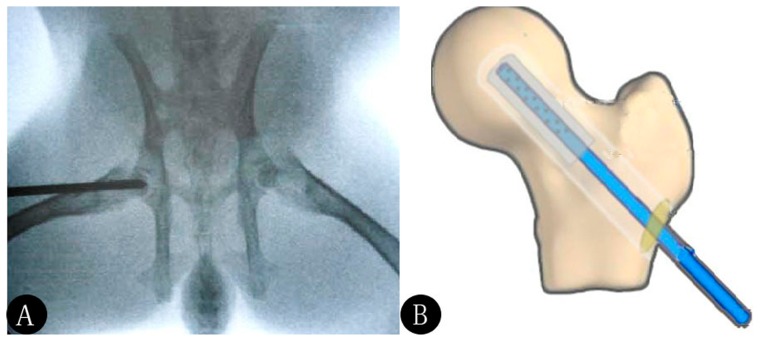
Core decompression surgery. An X-ray scan ensured the depth of decompression (A). A negative pressure connection tube was employed as shown in (B).
